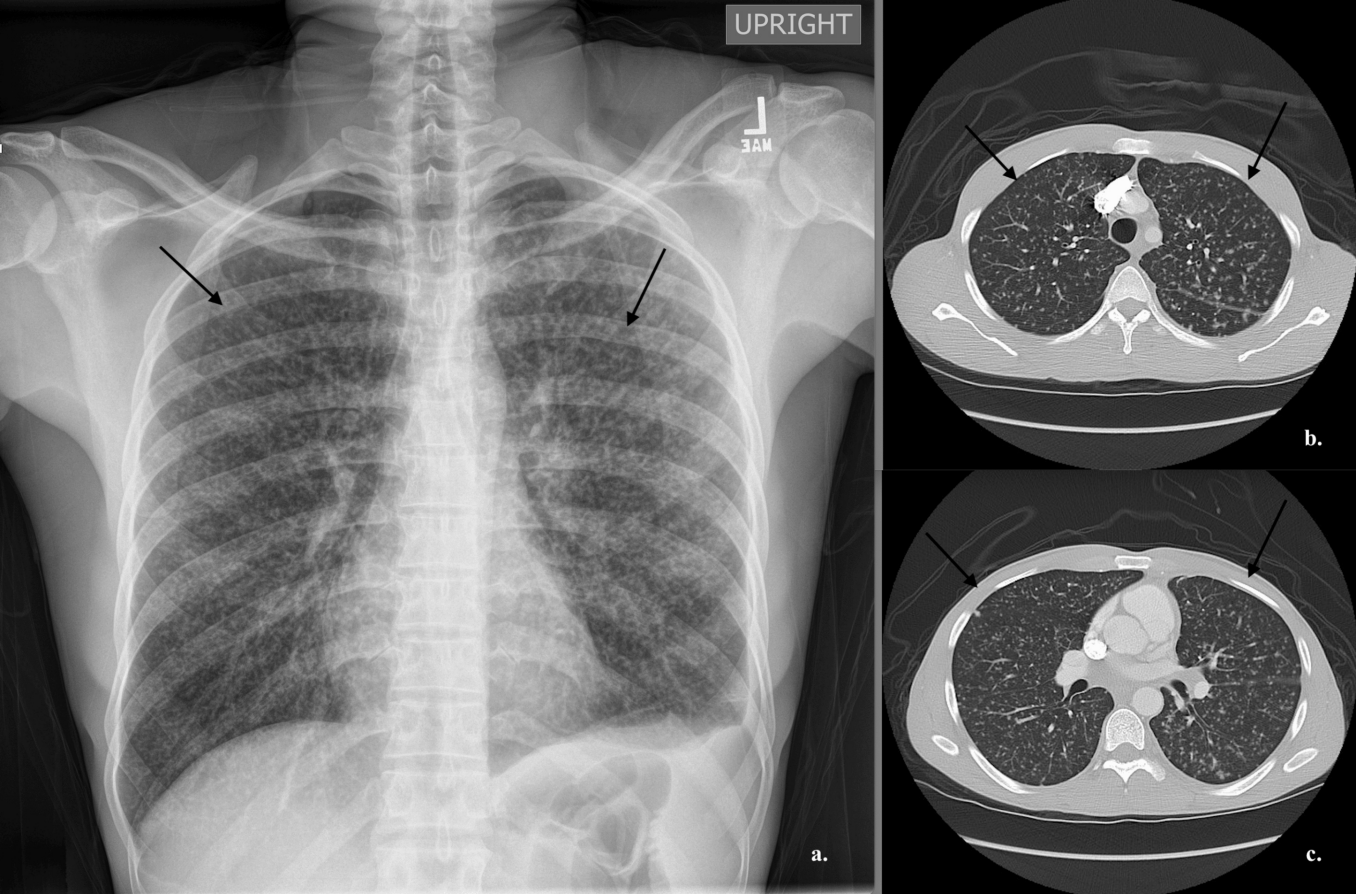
Chest X-ray and CT of chest. Both chest X-ray (a) and computer tomography (CT) chest (b and c) showed innumerable widespread bilateral miliary nodules suggestive of miliary tuberculosis.
